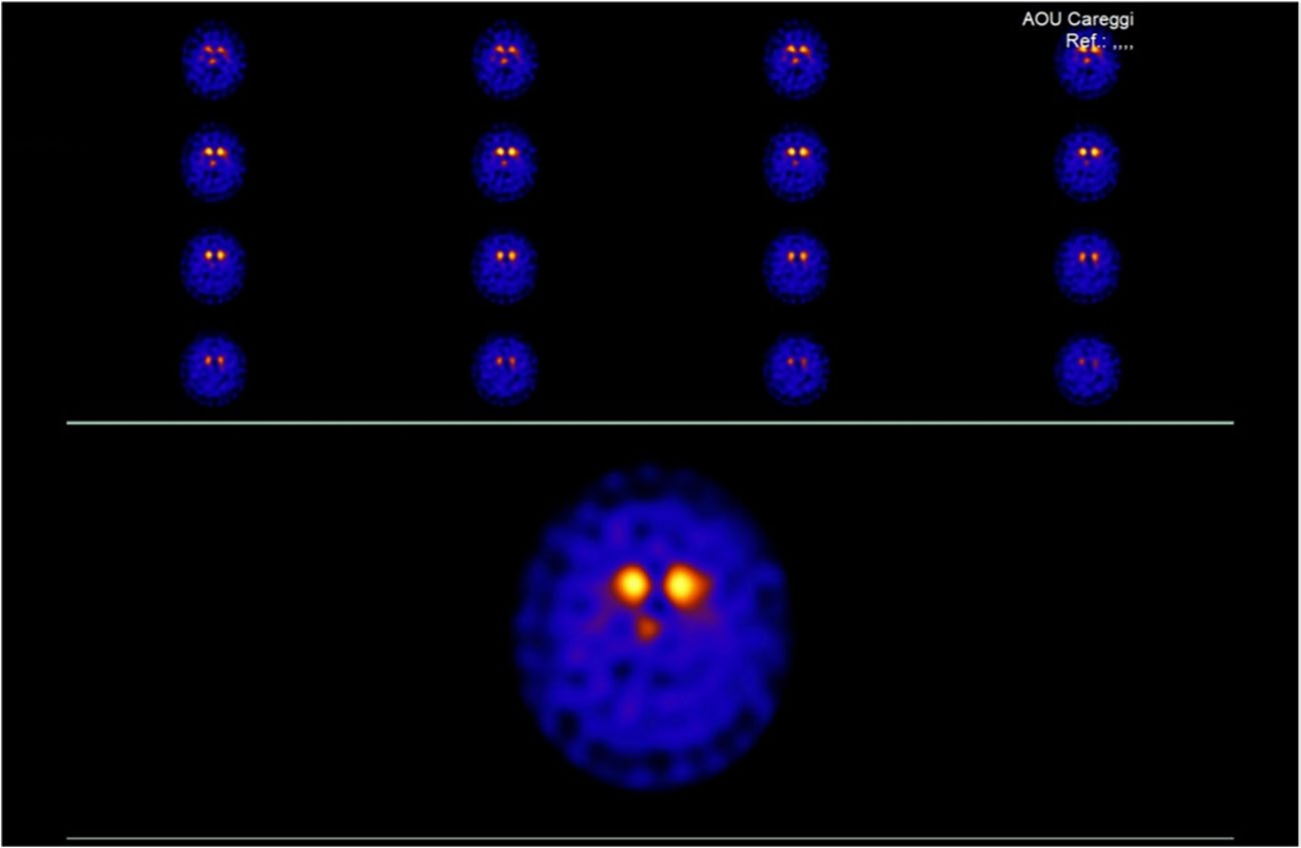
The dopamine transporter brain imaging with single-photon emission computed tomography (DAT-SPECT) revealing reduced uptake in both putamen nuclei, mostly in right putamen. Volume distribution (VD): right caudate 2.33, left caudate 2.35, right putamen 0.71, left putamen 0.88 (normal VD values: right caudate 2.58±0.41; left caudate 2.57±0.37; right putamen 2.14±0.41; left putamen 2.23±0.45)
